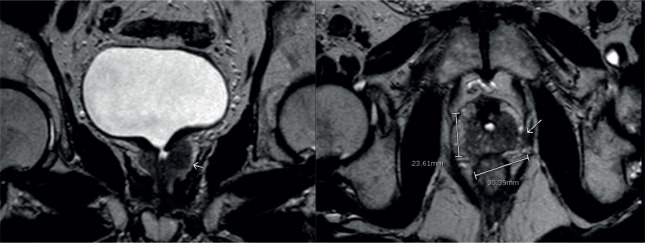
MRI shows an infiltrative tissue located in the left prostatic lobe. It is hypointense in the T2 sequence and enhances after endovenous contrast. It measures 30.3 × 23.6 mm.
